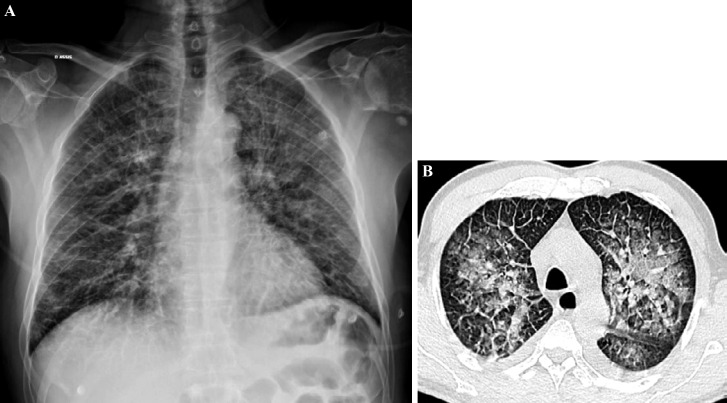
Patient âgé de 55 ans admis en réanimation pour prise en charge d'un syndrome pulmonaire à hantavirus compliqué d'une défaillance multiviscérale (respiratoire, rénale et hématologique) (crédit photo: M. Zappa) Légende: La radiographie pulmonaire (A) et le scanner thoracique (B) (fenêtre parenchymateuse passant par les lobes supérieurs) montrent des condensations alvéolaires et verre dépoli en plage prédominant dans les régions centrales associés à des lignes septales. Évolution favorable sous traitement symptomatique de réanimation et antibiothérapie. 55-year-old patient admitted in intensive care unit for management of a pulmonary hantaviral syndrome complicated by multivisceral failure (respiratory, renal and hematological) (photo credit: M. Zappa) Chest X-ray (A) and CT scan (B) (parenchymal window through the upper lobes) show alveolar condensations and ground glass predominantly in the central regions associated with septal lines. Favorable evolution under symptomatic resuscitation treatment and antibiotic therapy.
